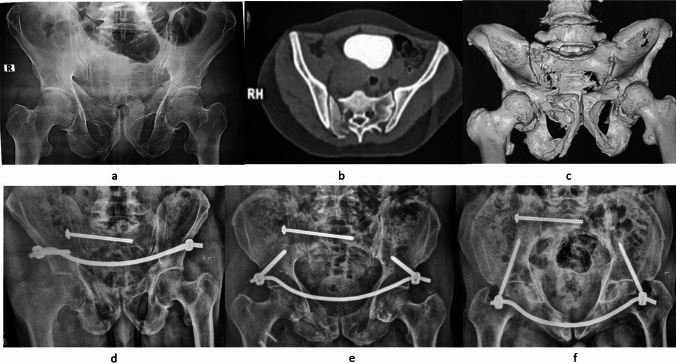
A LC 1 case with a tetra-ramic fracture. Anterior–posterior fixation was done. Preoperative AP X-ray a, axial CT b and 3D reconstruction c are shown. Follow-up at one year is shown in the outlet d, AP e and inlet f views. The patient healed with no secondary displacement
